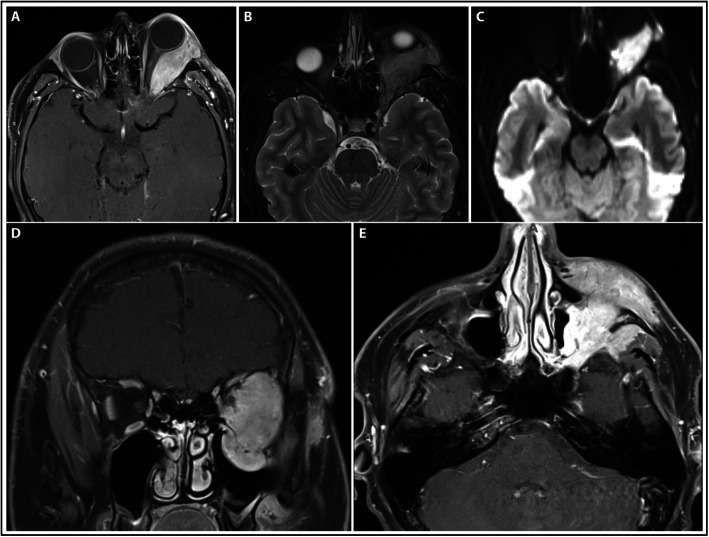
MRI brain and orbits after multiple biopsies and worsening of ophthalmic symptoms. a T1-weighted post-contrast axial sequence at the level of the optic nerve demonstrating avid enhancement of the left orbital lesion with infiltration of the left lateral rectus muscle and obvious proptosis without associated optic nerve enhancement. b T2-weighted axial sequence showing the lobulated left orbital mass to be hypointense and without significant surrounding edema. c Diffusion-weighted imaging showing significant lesional diffusion restriction. d Coronal and (e) additional axial T1-weighted post-contrast images showing extension of the homogeneously-enhancing mass into the left maxillary sinus, pterygopalatine fossa, infratemporal fossa, and surrounding soft tissues
